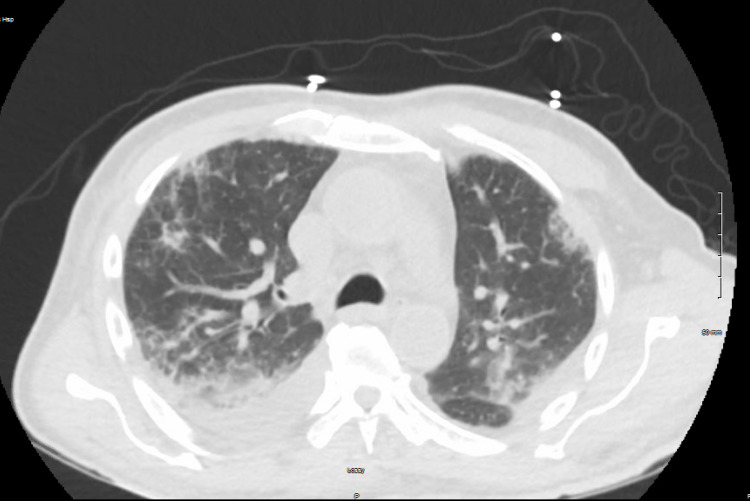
CT chest showing patchy bilateral interstitial and alveolar infiltrate with small bilateral pleural effusion
